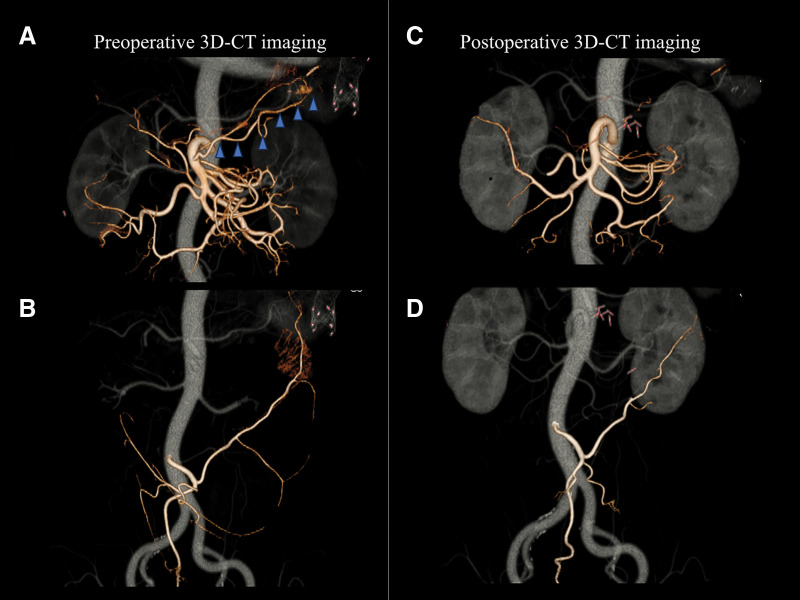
3D CT image reconstructed from contrast-enhanced CT. (A) Preoperative imaging revealed SMA-derived blood flow, with the AcMCA (arrowhead) serving as the main feeder to the tumor. (B) Preoperative imaging confirmed maintained perfusion from the IMA. (C) Postoperative imaging showing that blood flow from the AcMCA and Lt-MCA is no longer present. (D) IMA perfusion unchanged after surgery. AcMCA, accessory middle colic artery; IMA, inferior mesenteric artery; Lt-MCA, left branch of the middle colic artery; SMA, superior mesenteric artery
